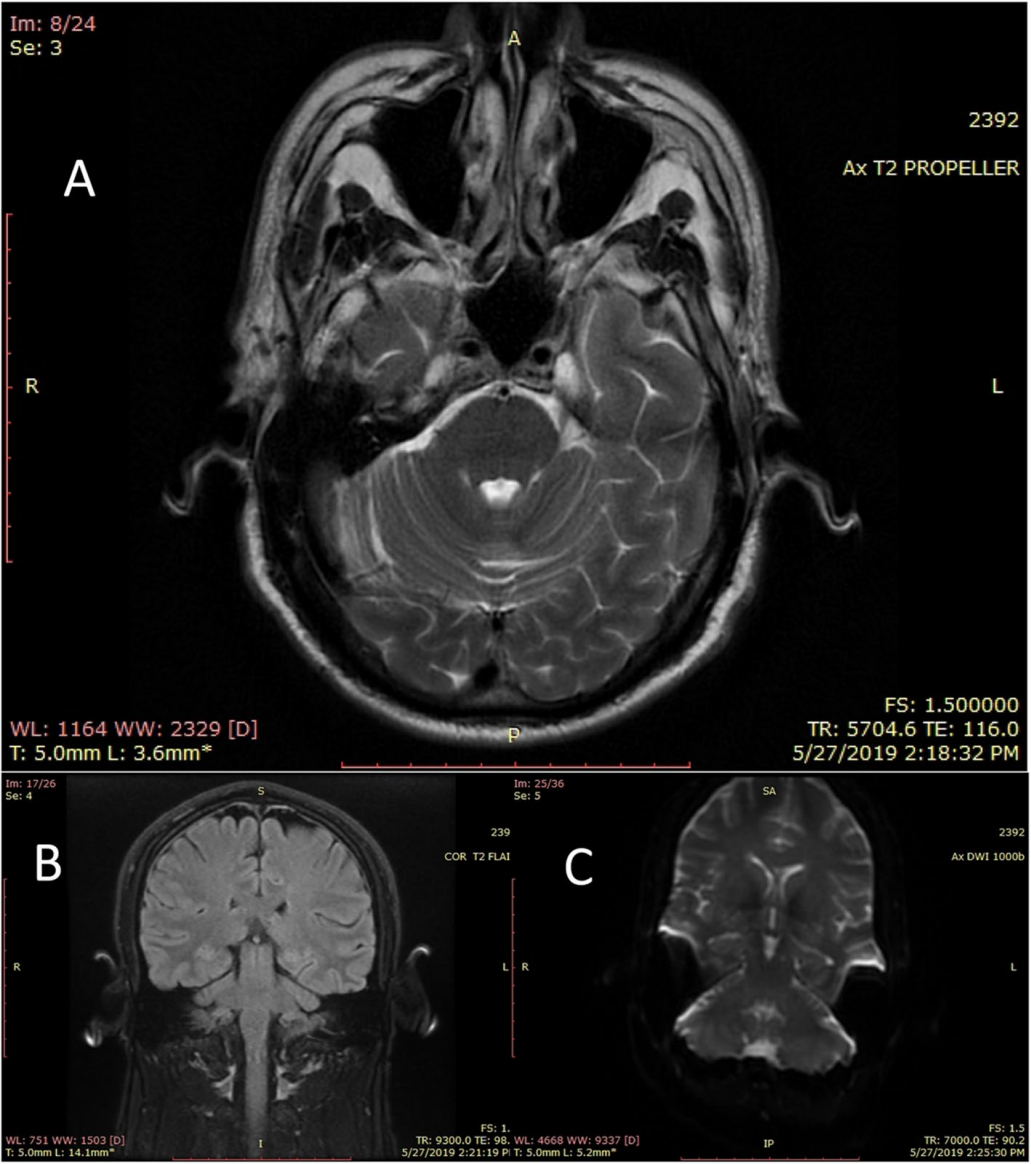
Sections of the MRI images of the patient at the brainstem level. A. T2 axial section. B. T2 flair coronal section. C. diffusion-weighted coronal section
